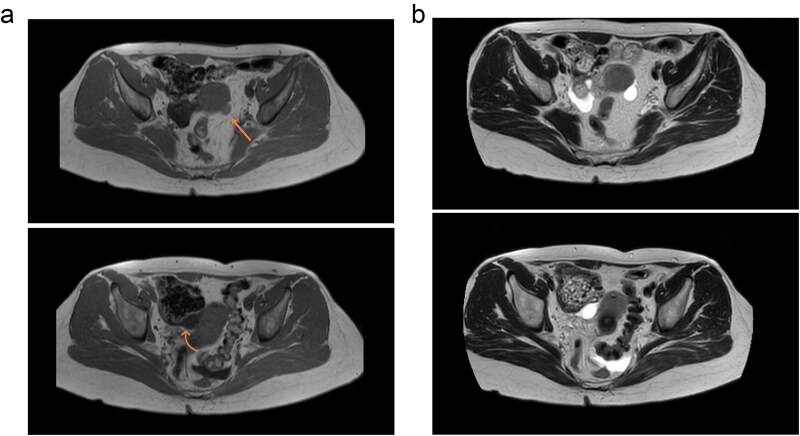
(a) Cystic lesions in T1-weightened MR images. Straight arrow indicates the cyst with haemorrhagic content, slightly hyperintense, whereas curved arrow the simple one. (b) Cystic lesions in T2-weightened MR images. In this sequence, the lesions are hyperintense.
